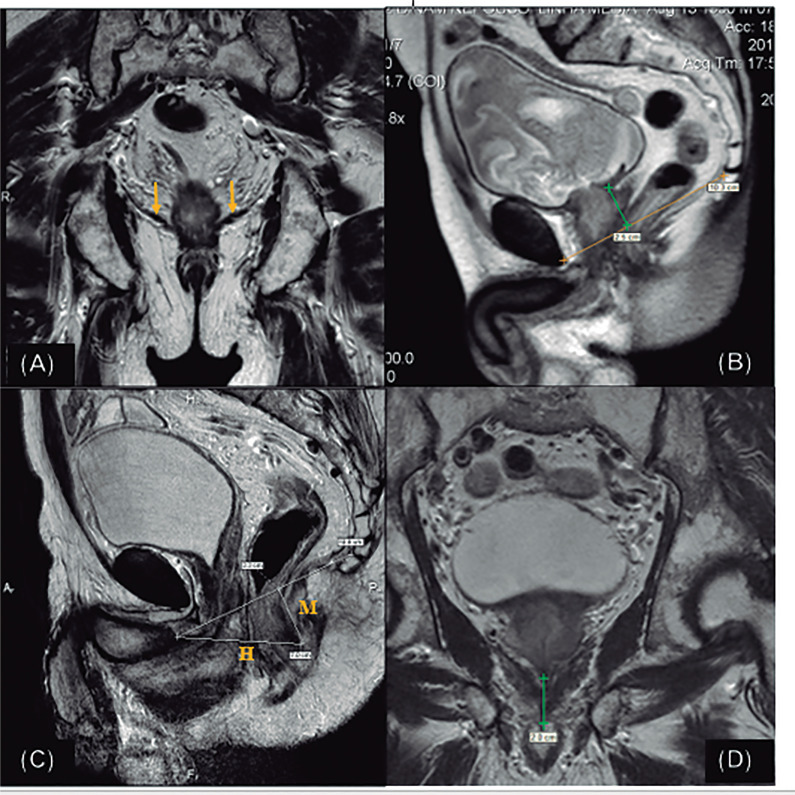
MRI standard measurements - A): Axial T2-weighted MR image shows LA thickness; B): Sagittal T2-weighted MR image shows the distance bladder to pubococcigeal line (DBPCL); C): Sagittal T2-weighted MR image shows the H and M lines; D): Coronal T2-weighted MR image shows the membranous urethra length (MU)
